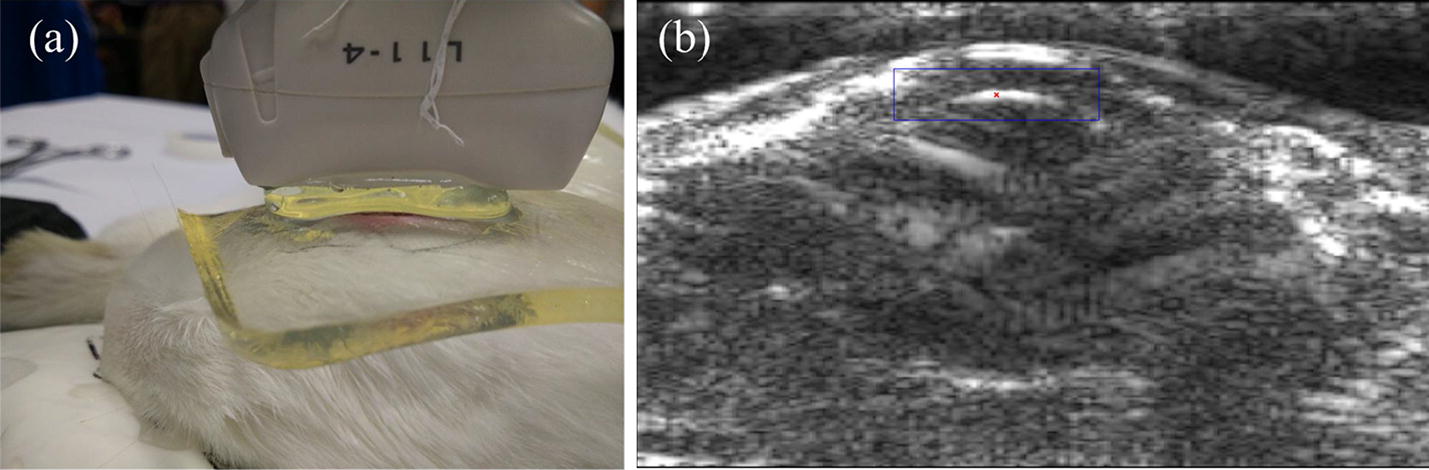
Measurement of lens: a Experimental measurement setup; and b a B-mode ultrasound image
